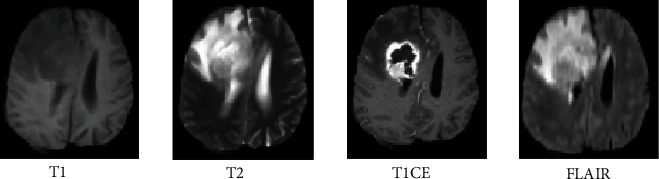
Four multisequence MR images.
